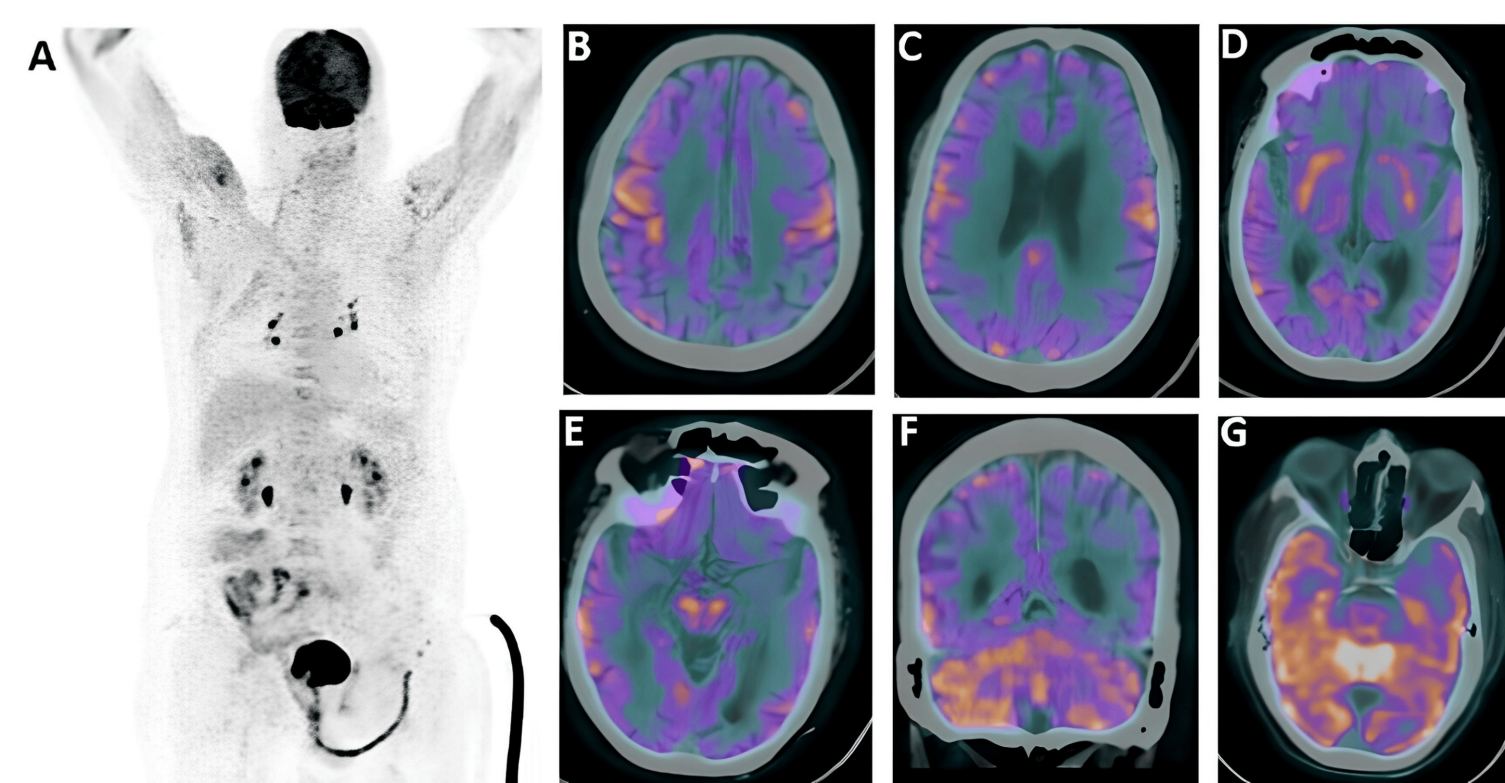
Maximum intensity projection (MIP) image of 18F-FDG PET/CT (A) showing physiological tracer uptake with no abnormal uptake. Fused PET/CT images show hypometabolism in bilateral frontal (B), bilateral parietal (C,D), and lateral temporal lobes (E) with hypermetabolism in the midbrain (E), bilateral cerebellum (F), and bilateral mesial temporal lobes (G). These findings are suspicious of autoimmune encephalitis.
